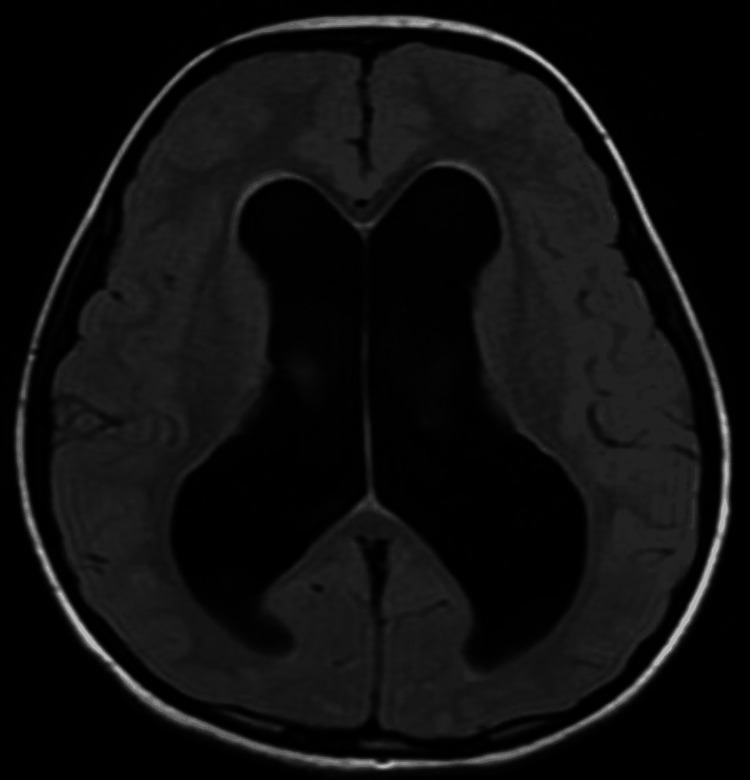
Brain MRI plain axial fluid-attenuated inversion recovery (FLAIR) image shows dilated bilateral lateral ventricles with generalised cerebral oedema.
